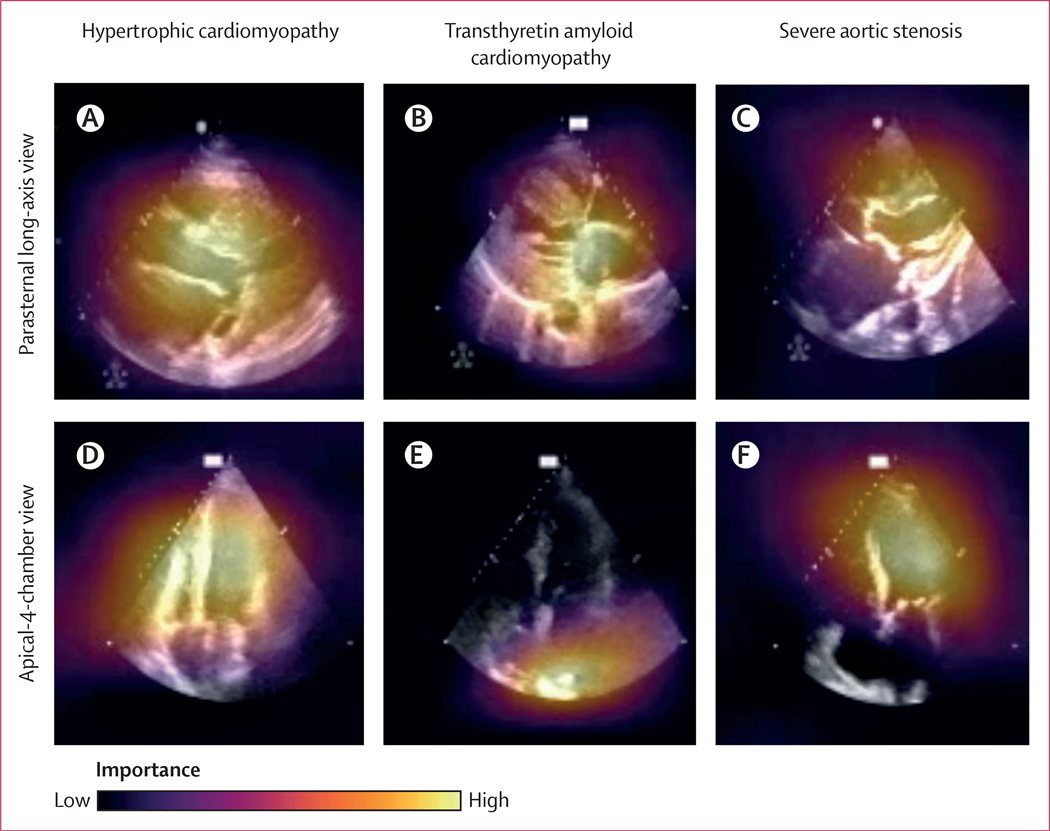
Saliency maps Activation maps for hypertrophic cardiomyopathy (A, B), transthyretin amyloid cardiomyopathy (C, D), and severe aortic stenosis (E, F) across parasternal long-axis and apical-4-chamber views obtained at the point of care in the emergency department. The colour scale denotes the relative importance of different areas, averaged across time, for each individual label.
